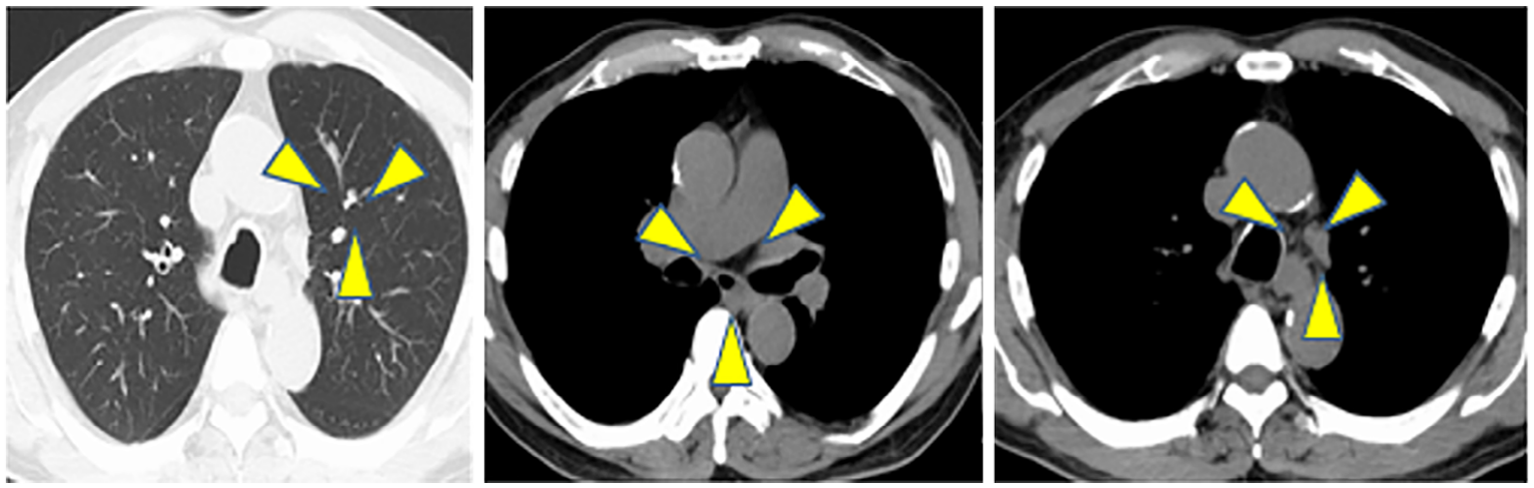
CT findings after 36 weeks of treatment for sicca syndrome caused by ICI treatment for mRCC in an elderly man. CT shows shrinkage of the metastatic lung nodule and mediastinal lymph nodes.
